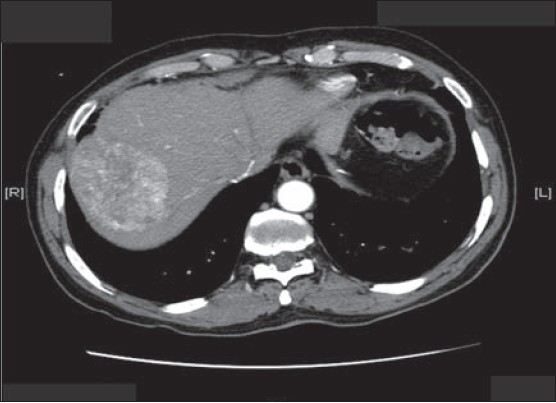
Triphasic CT scan of the abdomen showing a liver mass at segment 5 and 8; a) arterial phase, b) portal-venous phase.
